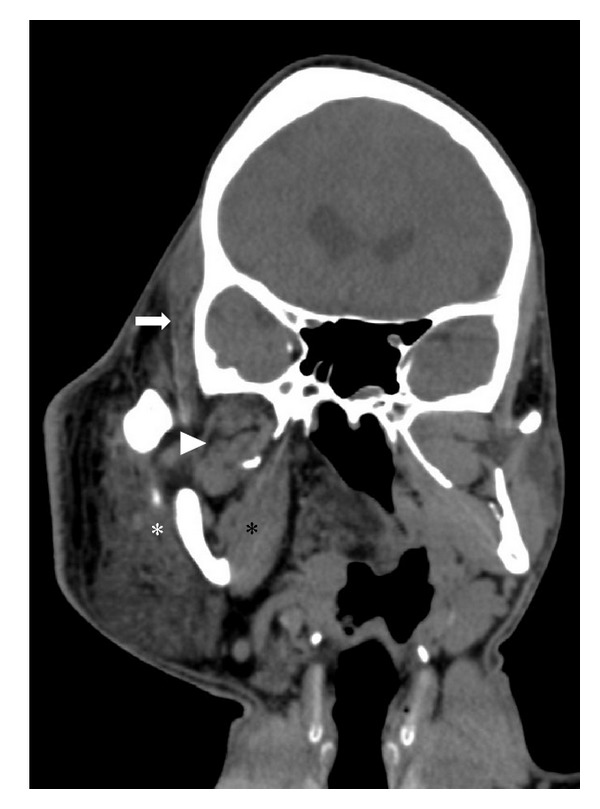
Coronal CT scan revealing hyperplasia of the right mandibular condyle, medial pterygoid (black asterisk), lateral pterygoid (arrowhead), masseter (white asterisk), and temporalis muscle (white arrow).
